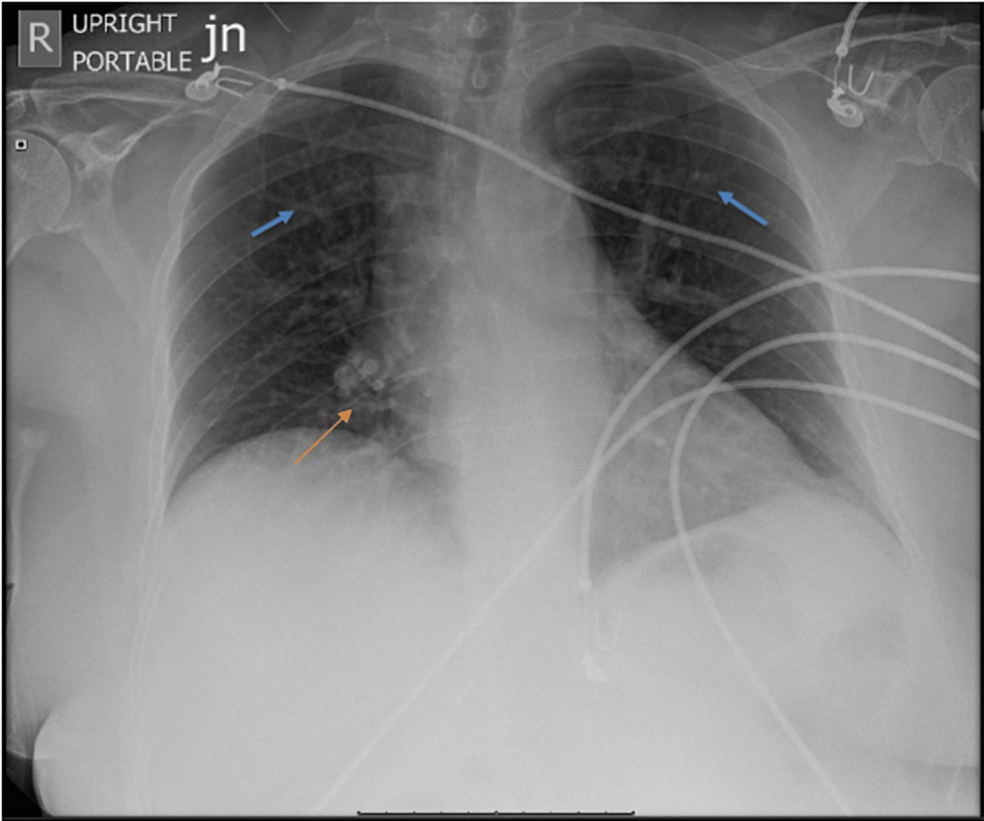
Chest X-ray showing a normal cardiac silhouette Some calcification is present in the right hilar region (orange arrow) and aortic knob. There is a slight prominence of vascular markings (blue arrows)
